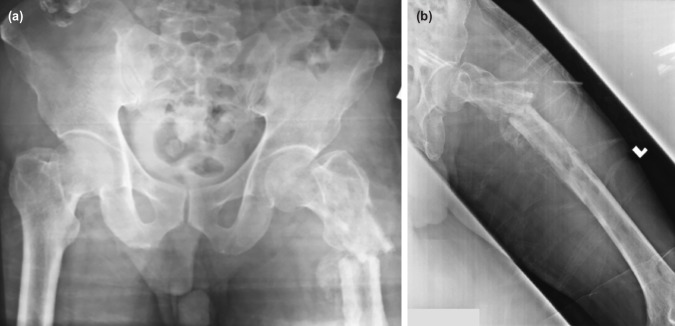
Mr. N, a 63-year old male, with left thigh pain after trivial fall. Radiographs (a) and (b) showing diffuse lytic lesion over the whole length of left femur with pathological subtrochanteric fracture.
